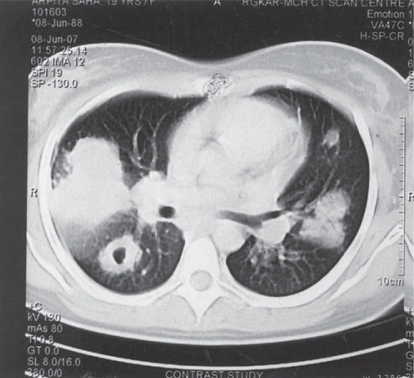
CT scan of thorax (mediastinal view) showing bilateral multiple pulmonary nodules of varying size with cavitation in one nodule with no mediastinal lymphadenopathy.
